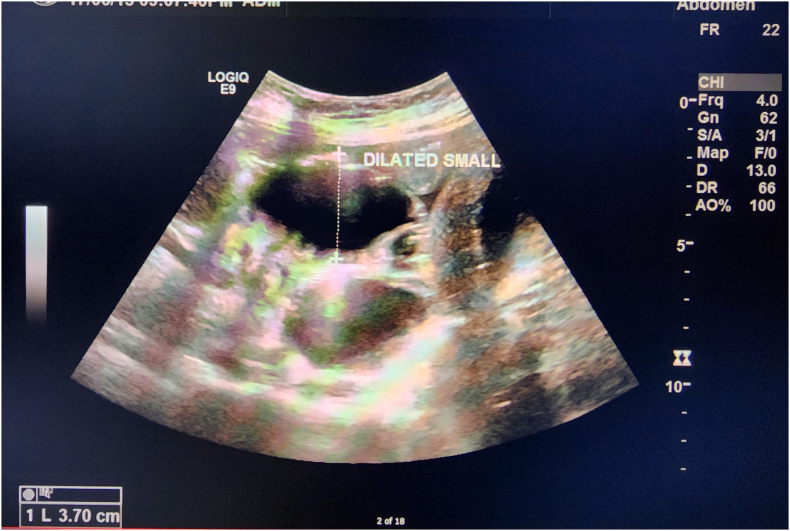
Ultrasound abdomen-showing dilated small bowel loops with free fluid in abdomen.
